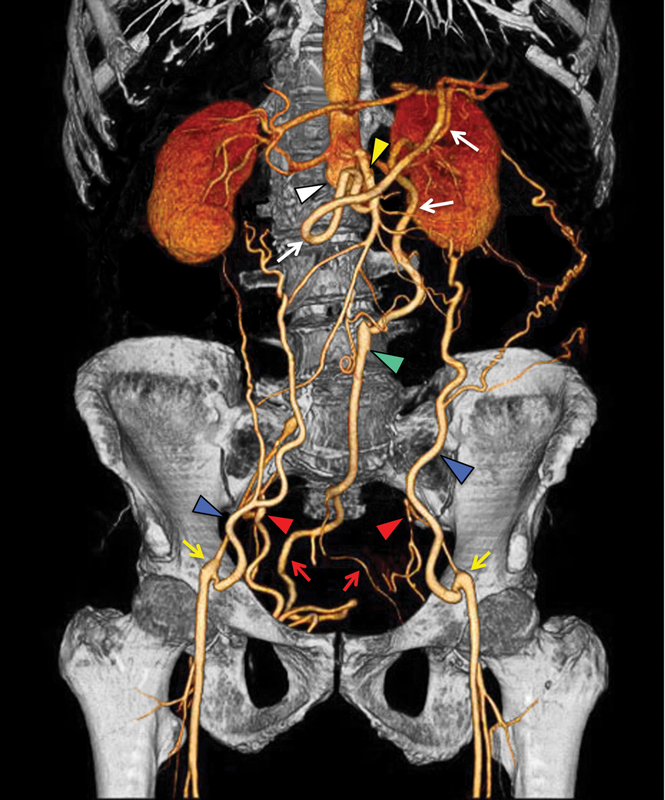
Multidetector computed tomography with coronal volume rendering reconstruction shows abdominal aortic occlusion below renal arteries origin's (white arrowhead) with extension to bilateral common iliac arteries. Note the hypertrophic network of collateral vessels: (1) superior mesenteric artery (yellow arrowhead) communicates with inferior mesenteric artery via Riolan's arc (white arrows), (2) inferior mesenteric artery (green arrowhead) through the superior rectal artery (red arrows) provides blood flow to internal iliac artery (red arrowheads), and (3) inferior epigastric arteries (blue arrowheads) guarantee blood flow to the bilateral external iliac arteries (yellow arrows).
